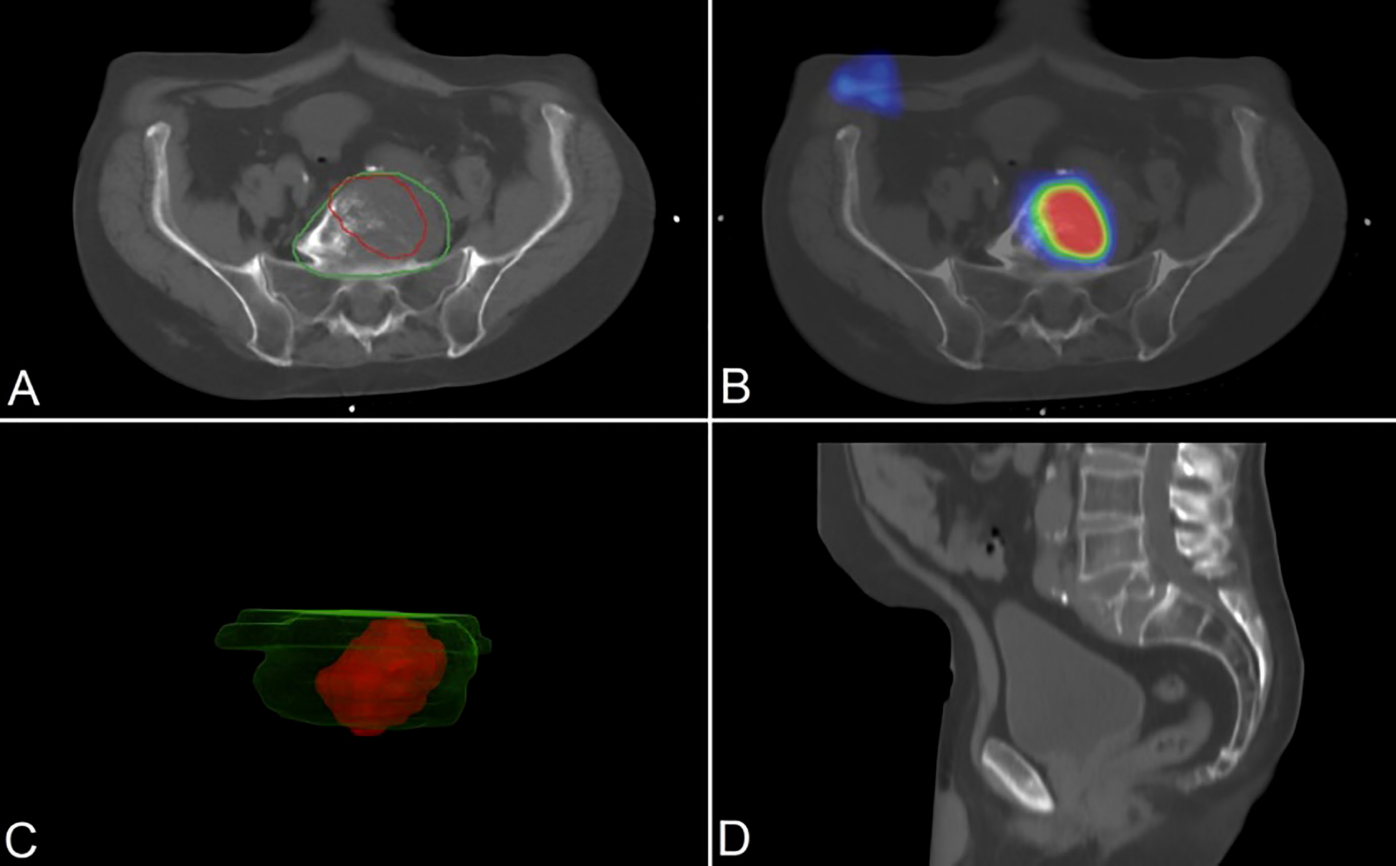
A 74-year-old patient with PSMA-positive lytic vertebral metastasis (PSA 90.68 ng/ml; Gleason: 3 + 4 = 7). Topometric CT (axial (A) and sagittal (D) slices) fused and registered with ^99m^Tc-PSMA-SPECT images (axial slice (B)). Smoothed 3D polygon model of the GTV_CT_ (green outlines) and BTV_PSMA_ (red outlines) (C). PSMA, prostate-specific membrane antigen; PSA, prostate-specific antigen; SPECT, single-photon emission computed tomography; GTV_CT_, gross tumor volume on the topometric CT; BTV_PSMA_, biological target volume on the PSMA-based examination.
